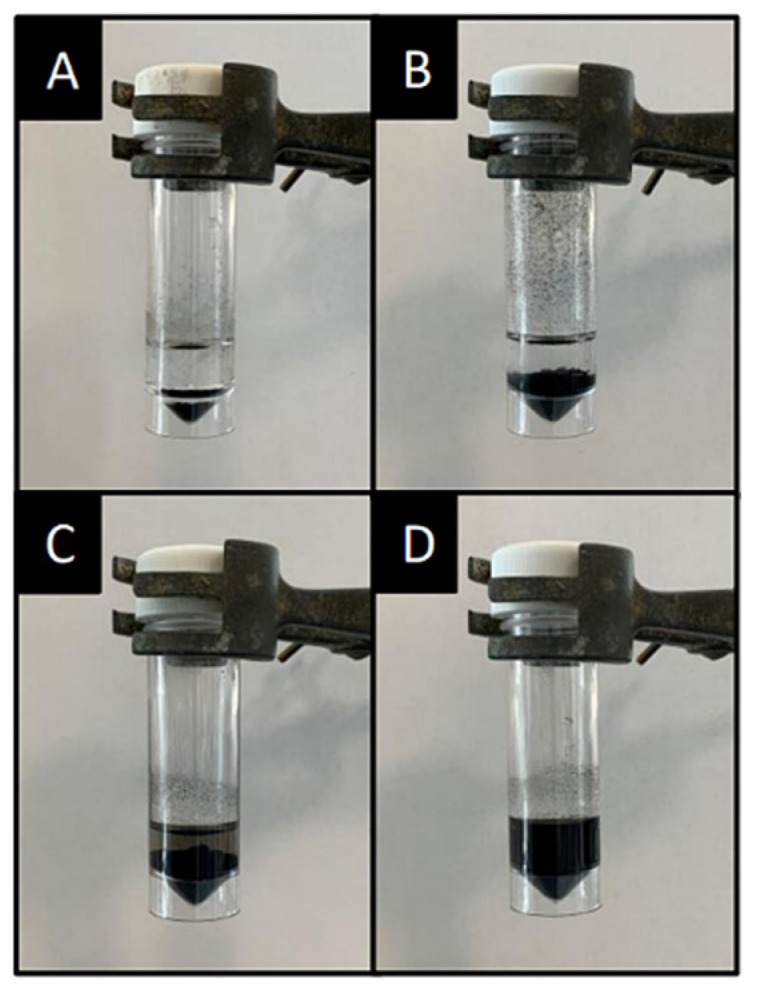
MWCNT suspensions in 10 mL of (A) dH_2_0, (B) 100% ethanol, (C) MES buffer, and (D) diamine oxidase enzymatic solution after ultrasonication for 20 min and left undisturbed for 2 h.
